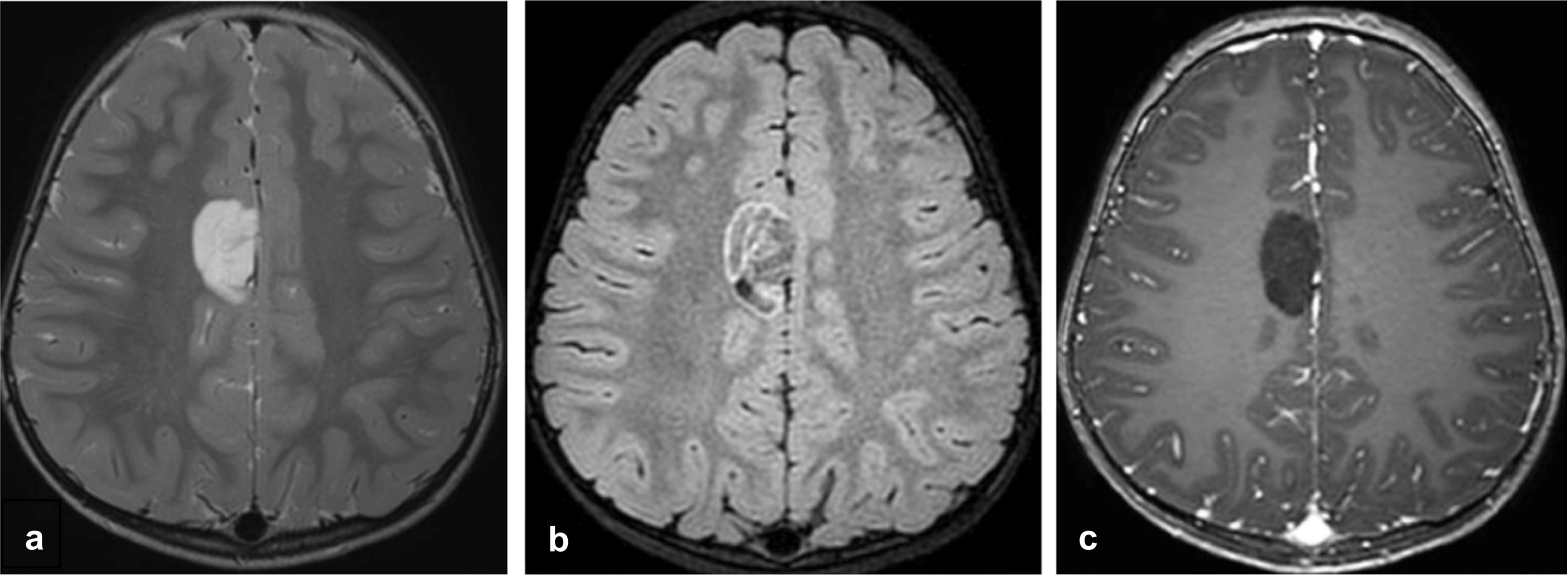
DNT with *PDGFRA* mutation in a 3-year-old female presenting with a seizure. Axial T2-weighted (a), T2 FLAIR (b), and T1-weighted contrast-enhanced (c) MR images demonstrate a “bubbly,” cortically-based, non-enhancing right paramedian frontal lobe mass with “T2-FLAIR mismatch” sign
